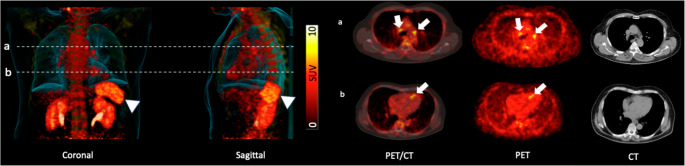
CXCR4-targeted ^68^Ga-PentixaFor PET/CT in a patient after acute myocardial infarction. Dotted lines on the PET/CT maximum intensity projections (MIP, left) in a coronal and sagittal view indicate trans-axial slides on the right. On trans-axial PET/CT and PET in (a), an intense PET signal can be observed in mediastinal lymph nodes (arrows), which was not enlarged on the CT portion. In (b), the infarct area in the myocardium also exhibits intense in-vivo CXCR4 expression (PET/CT, PET, arrows), while there is no respective finding on CT. On the MIPs (left), radiotracer signal can also be observed in the bone marrow and in the spleen (arrowheads). SUV=standardized uptake value
